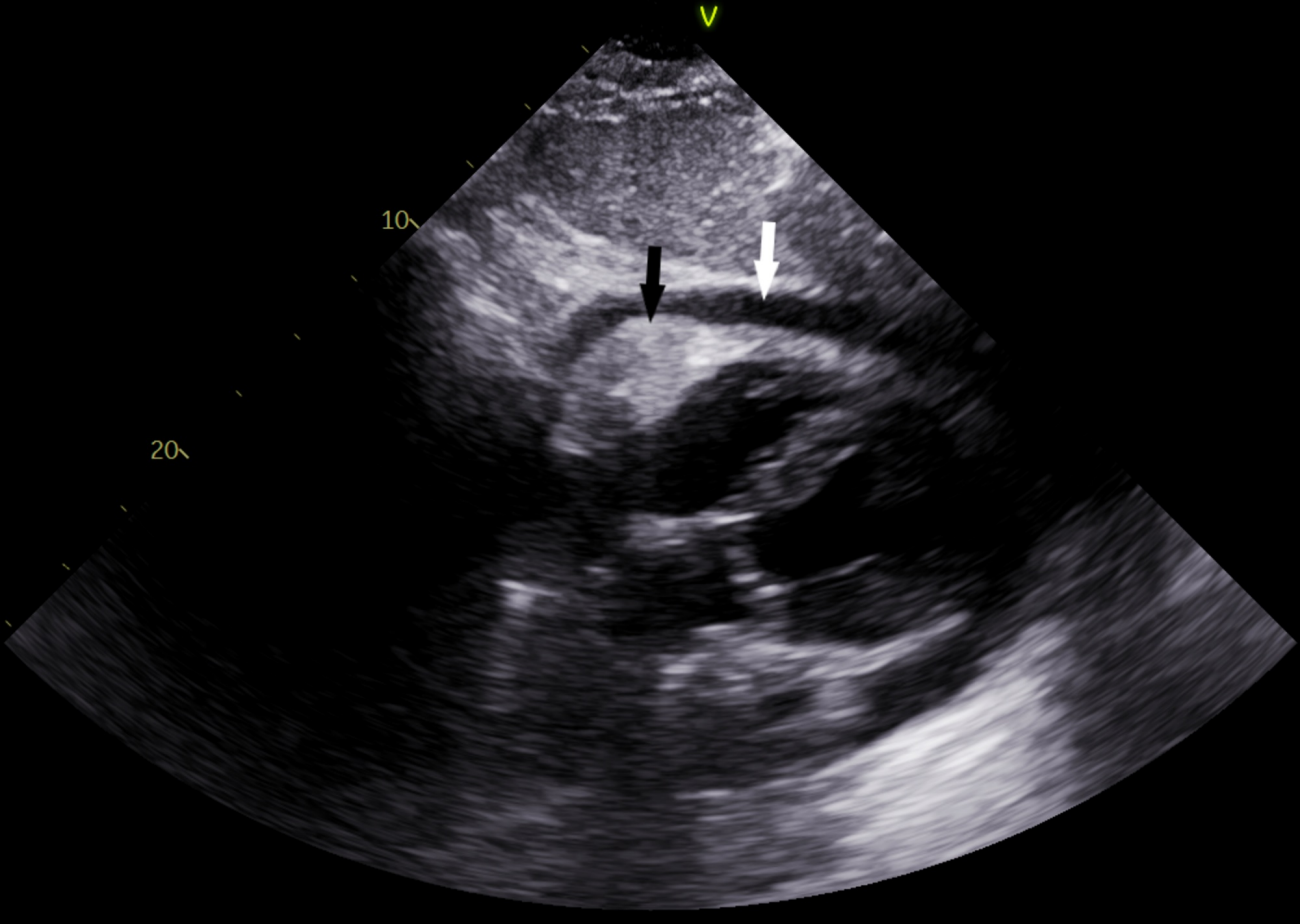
Transthoracic echocardiogram Pericardial effusion (white arrow) can be seen, along with organized component of the effusion (black arrow).
